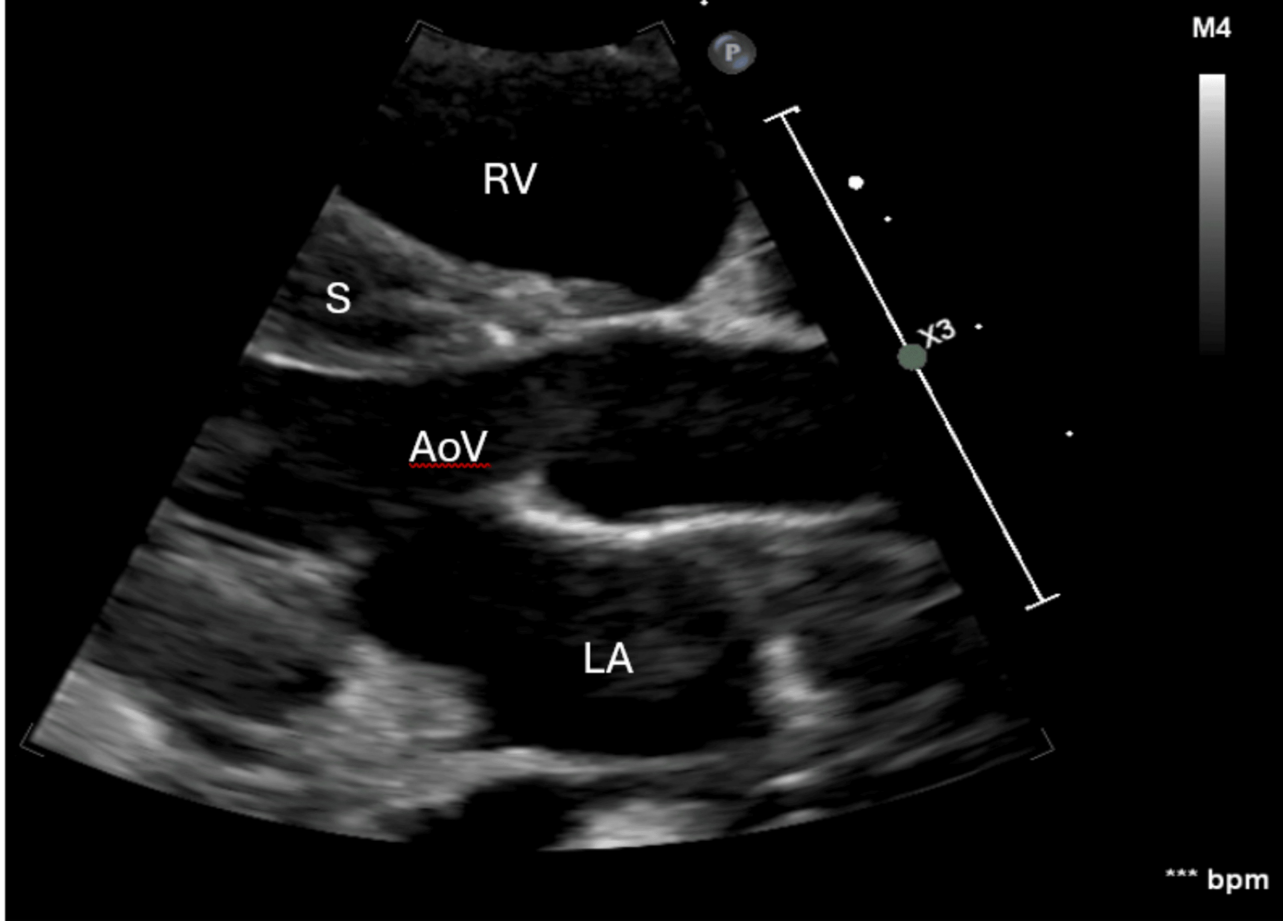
Echocardiogram showing thrombus resolution RV: right ventricle, S: septum, AoV: aortic valve, LA: left atrium
